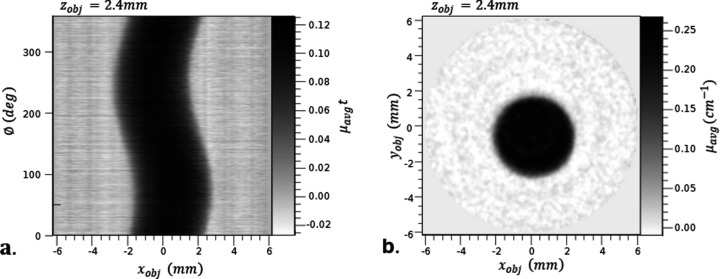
Sinogram (*a*) and axial view (*b*) of a CT scan of a nylon screw taken with the compressed beam produced by the parallel-beam compressor.
